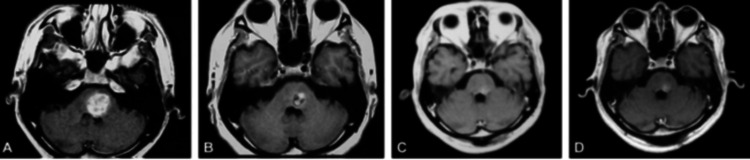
Evolution of a brainstem cavernoma treated with stereotactic radiosurgery after 36 months of follow-up. A: MRI imaging of the brain in axial planes in T1 sequence showing a brainstem cavernoma after stereotactic radiosurgery; B: Follow-up MRI imaging in T1 axial planes showing the brainstem cavernoma at 12 months post stereotactic radiosurgery; C: Follow-up MRI imaging in T1 axial planes at 24 months post stereotactic radiosurgery showing a significant volume reduction; D: Follow-up MRI imaging in T1 axial planes 36 months post stereotactic radiosurgery showing a stable lesion with no volume increase.
